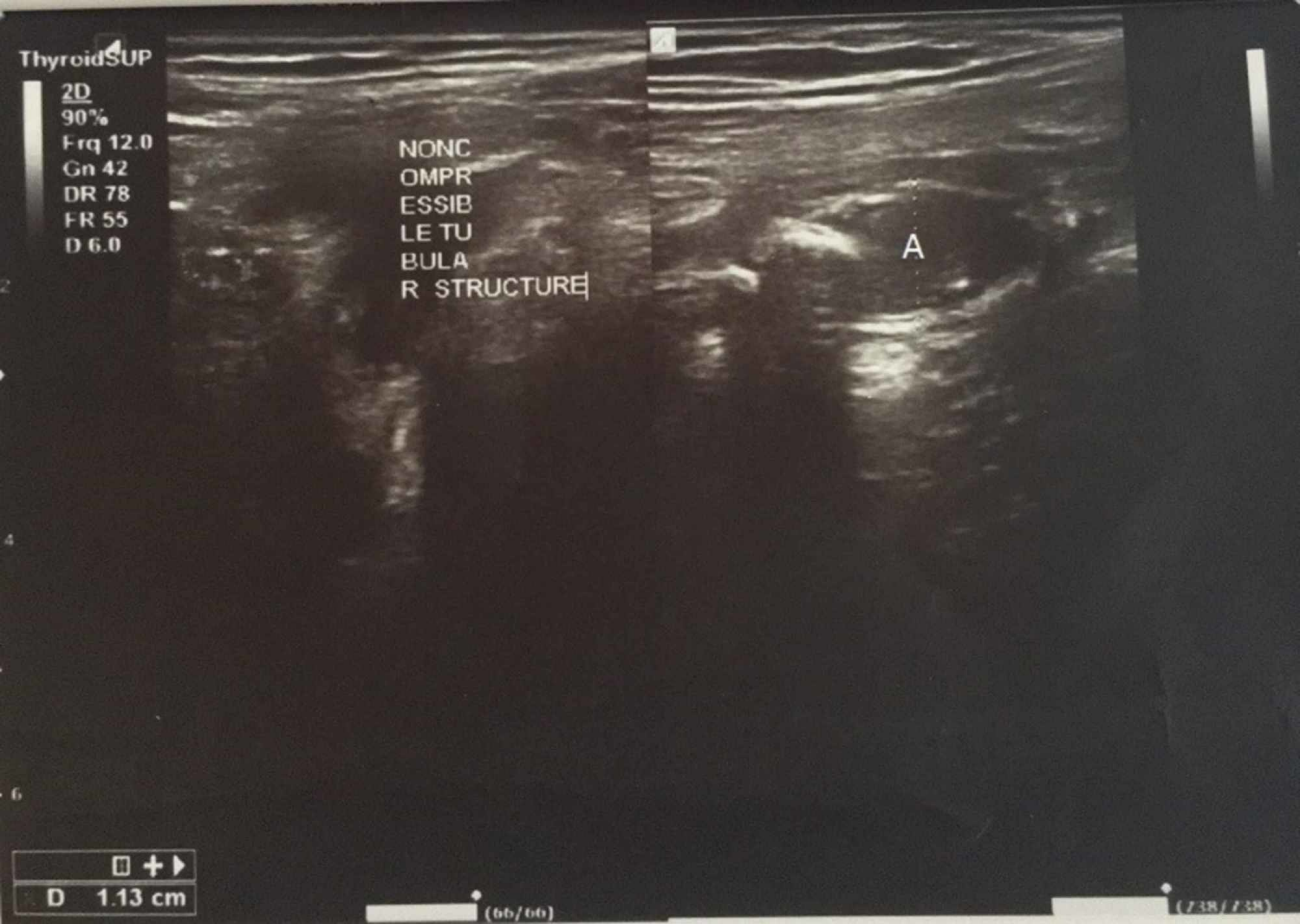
Ultrasonography of the abdomen showing a noncompressible blind-ended tubular structure without periappendiceal fluid (A)
